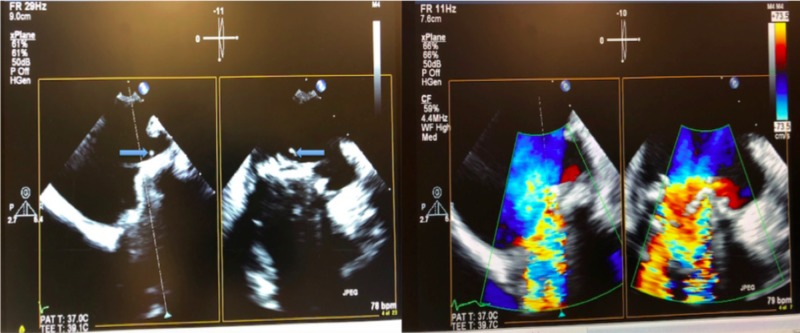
(Left) Mobile friable vegetations arising from the mitral prosthetic valve. (Right) Severe mitral regurgitation with torrential back flow across the prosthetic mitral valve demonstrated by Colour Doppler.
